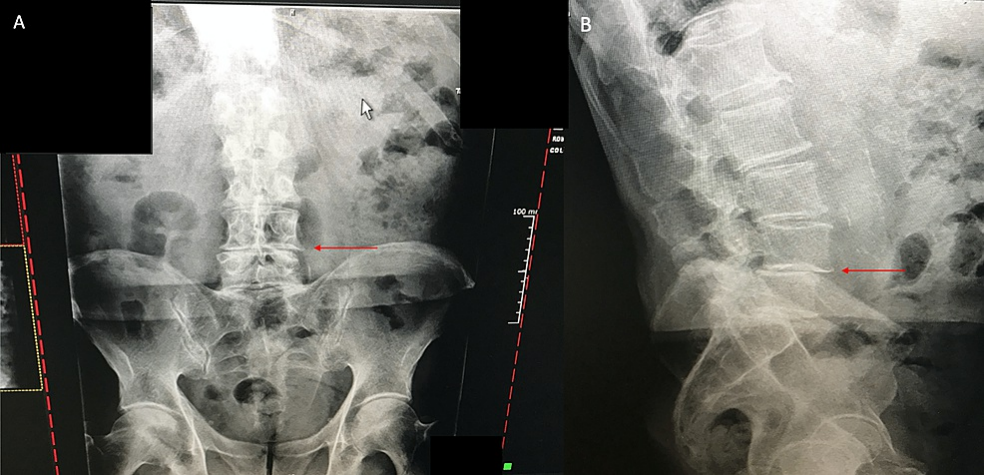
Patient lumbar X-rays. Standing lumbar AP view (A) and lateral view (B). The red arrow indicates L4-5 disc degeneration with grade 1 spondylolisthesis. AP: anterior-posterior.
